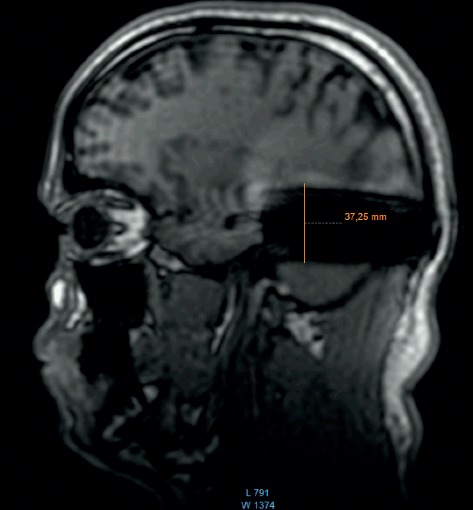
MRI survey pattern of the sagittal plain of a 1.5 T scanning of an Oticon ZTI Implant.
